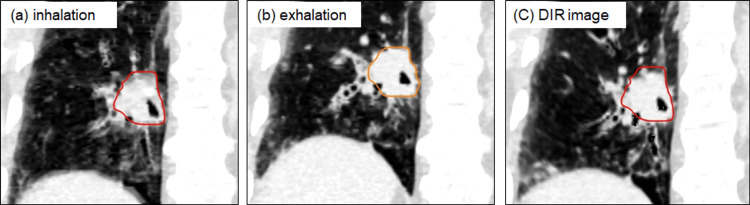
Clinical case (a) Inhalation and (b) exhalation CT images and (c) the deformable image registration (DIR) results using the B-spline.
